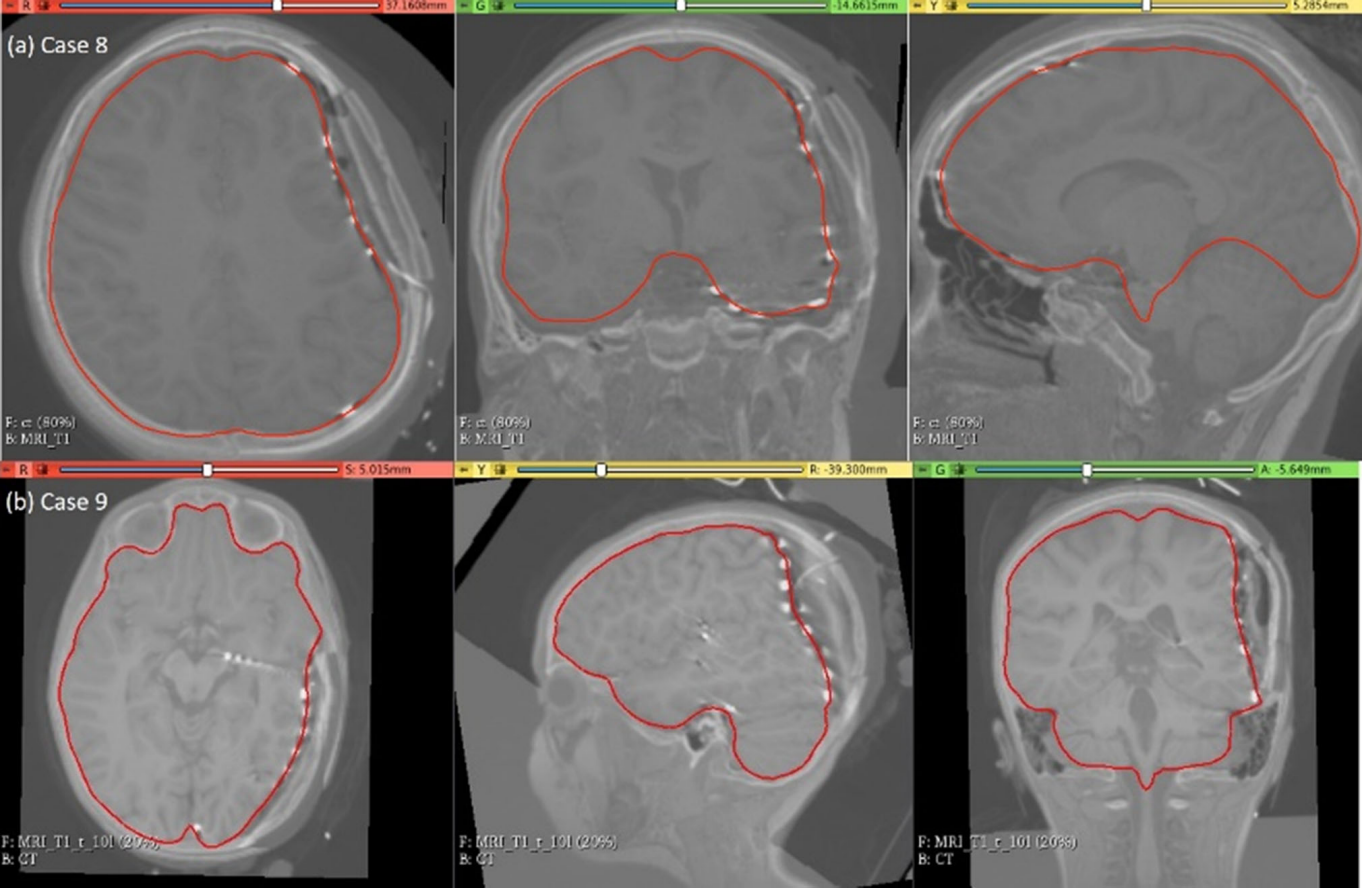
Visualisation of predicted intra-operative MRIs for case 8 and case 9 registered on CT with implanted intra-cranial electrodes along with predicted deformed brain model surface (red line)
